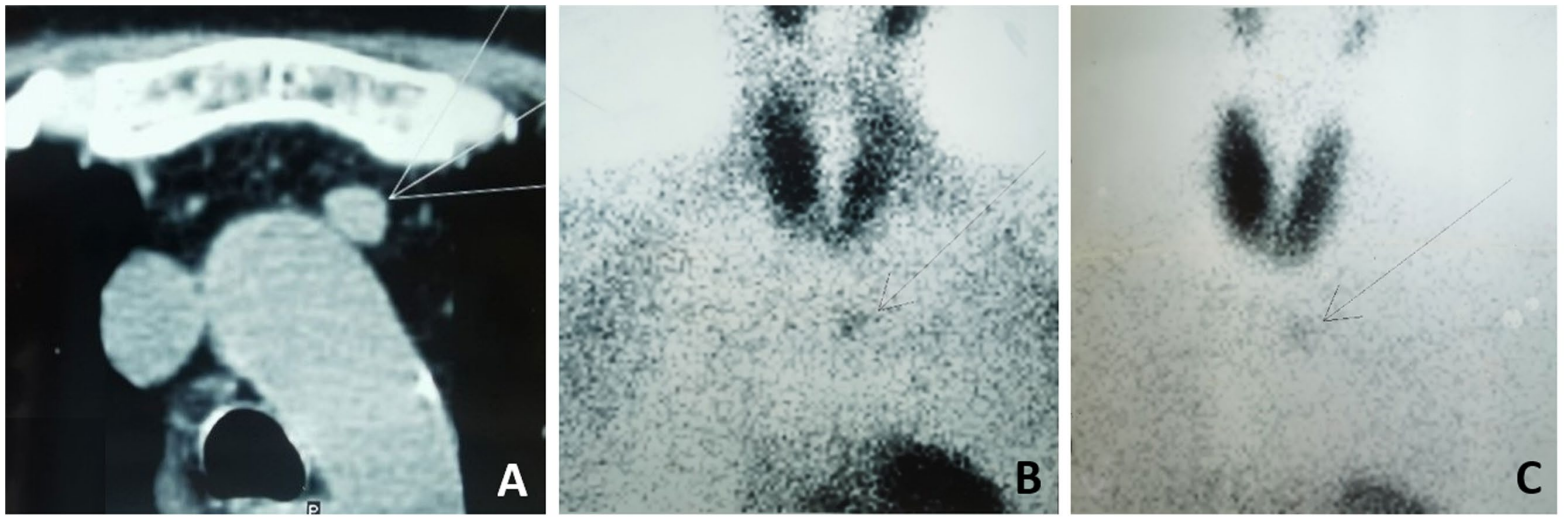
Chest CT scan and ^99m^Tc-MIBI before the 1st surgery. (A) Chest CT showed a 12 x11-mm nodule adjacent to aortic arch. (B)
